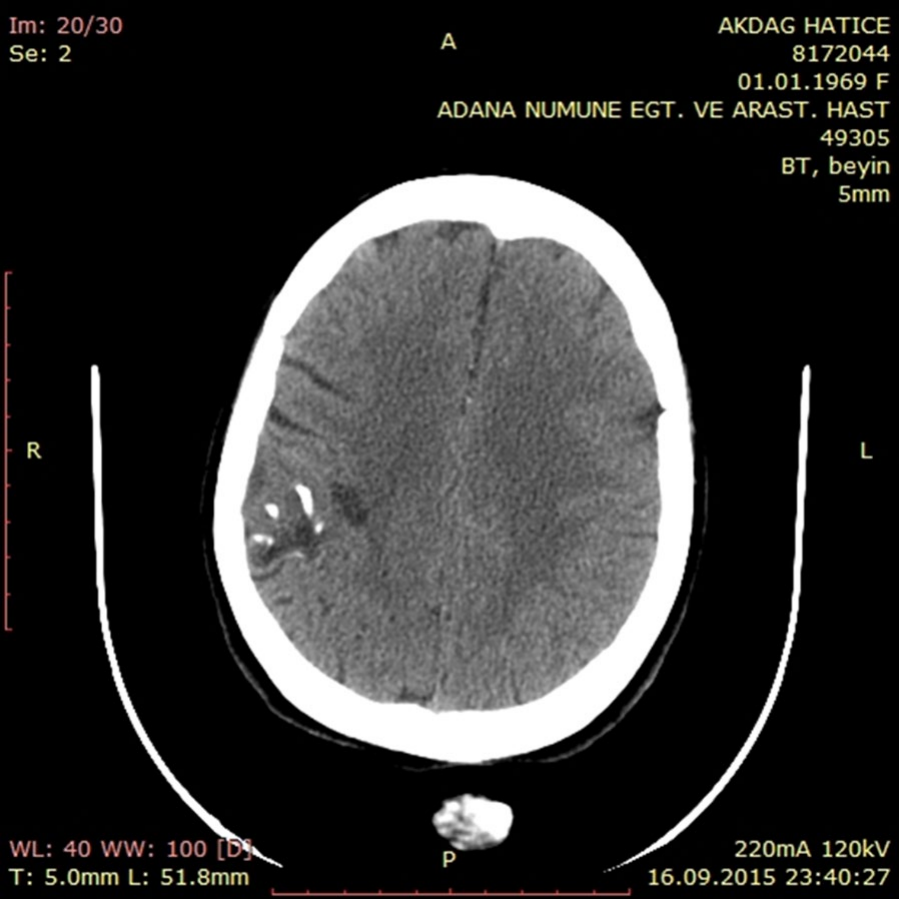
Preoperative cranial CT image of the patient
